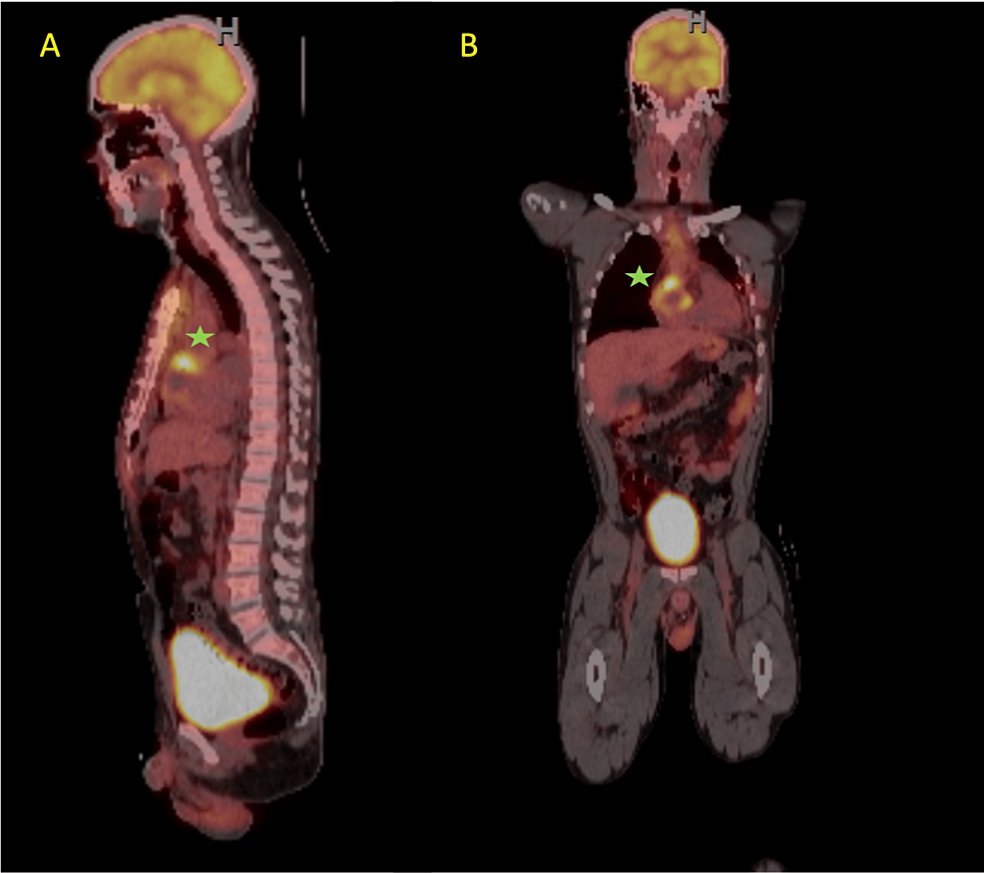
PET 1630 FDG with a focus of severe increased FDG concentration (Star*) with a relatively large central focal defect localized on the free wall of the right atrial area of the myocardium. FDG: fluorodeoxyglucose PET: positron emission tomography
